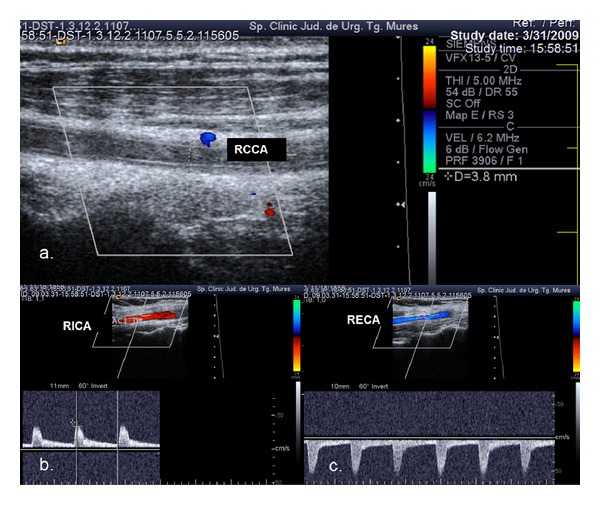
Ultrasound examination showing hypoplastic and occluded right CCA. (a) Colour mode examination: RCCA occlusion, no flow in the right common carotid artery, the vessel lumen is filled with thrombotic material, and the vessel diameter is 3.8 mm. (b), (c) Duplex mode examination: anterograde flow in the right ICA and retrograde flow in the right ECA.
